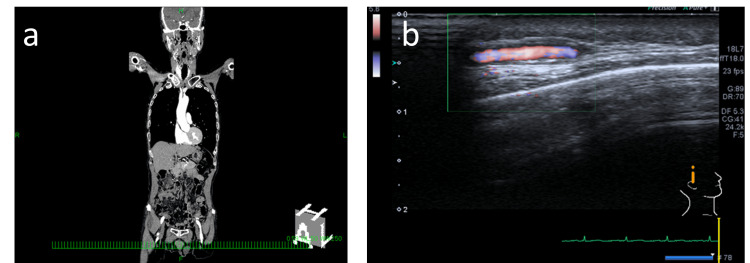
Images of (a) Whole-body contrast-enhanced computed tomography, and (b) Temporal artery ultrasonography These findings did not suggest vasculitis.
